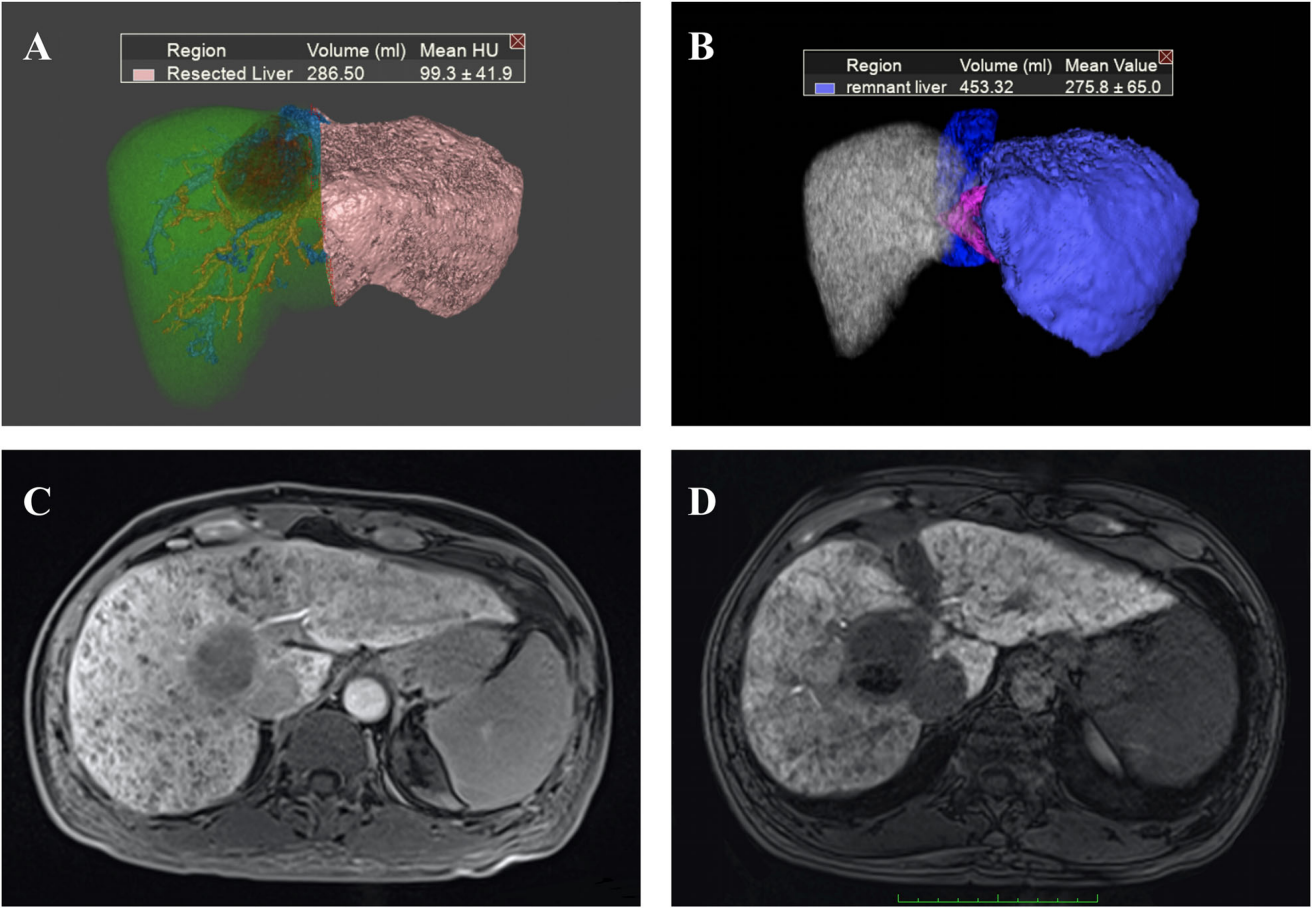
Interstage liver growth and liver function assessment. A A 48-year-old male patient, who underwent ALPPS stage 2 surgery after the MRI evaluation of the left lobe volume and function. Preoperative three-dimensional CT and virtual right hemi-hepatectomy show the liver volume of the left lobe is 286.50 mL (22.66% of the standard liver volume). B Three-dimensional MRI in 78 days after ALPPS stage 1 shows the liver volume of the left lobe is 453.32 mL (35.84% of the standard liver volume). C Axial MRI in hepatobiliary phase in 9 days after ALPPS stage 1 shows liver cirrhosis and dysfunction of the liver parenchyma: heterogeneous contrast enhancement with moderate intensity. D Axial MRI in hepatobiliary phase in 78 days after ALPPS stage 1 shows improved liver function, particularly in the left lobe: persistent heterogeneous parenchymal contrast uptake, but with significantly intensified enhancement (and increased volume as demonstrated on B), the patient proceeds to stage 2 soon after the examination
